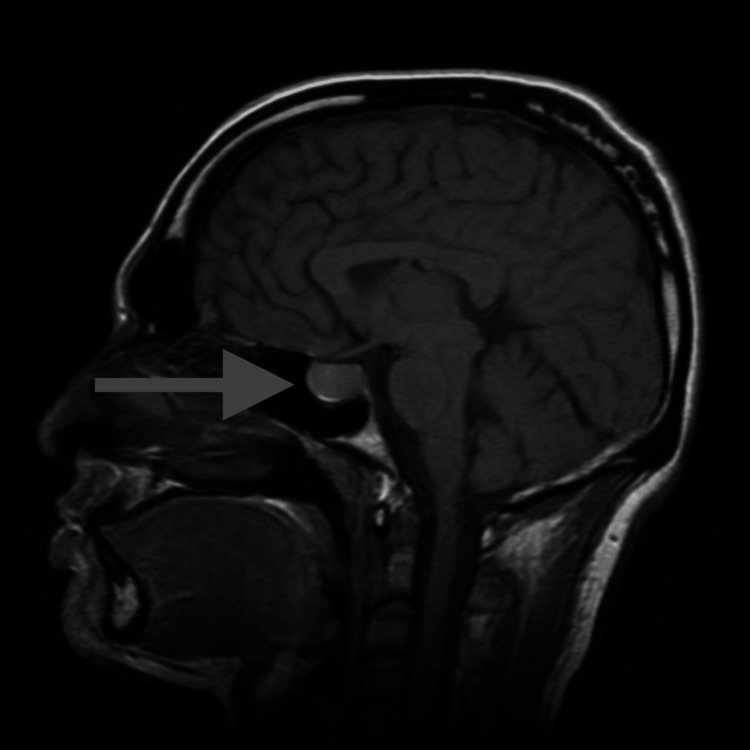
MRI showing cystic adenoma before cabergoline treatment.
